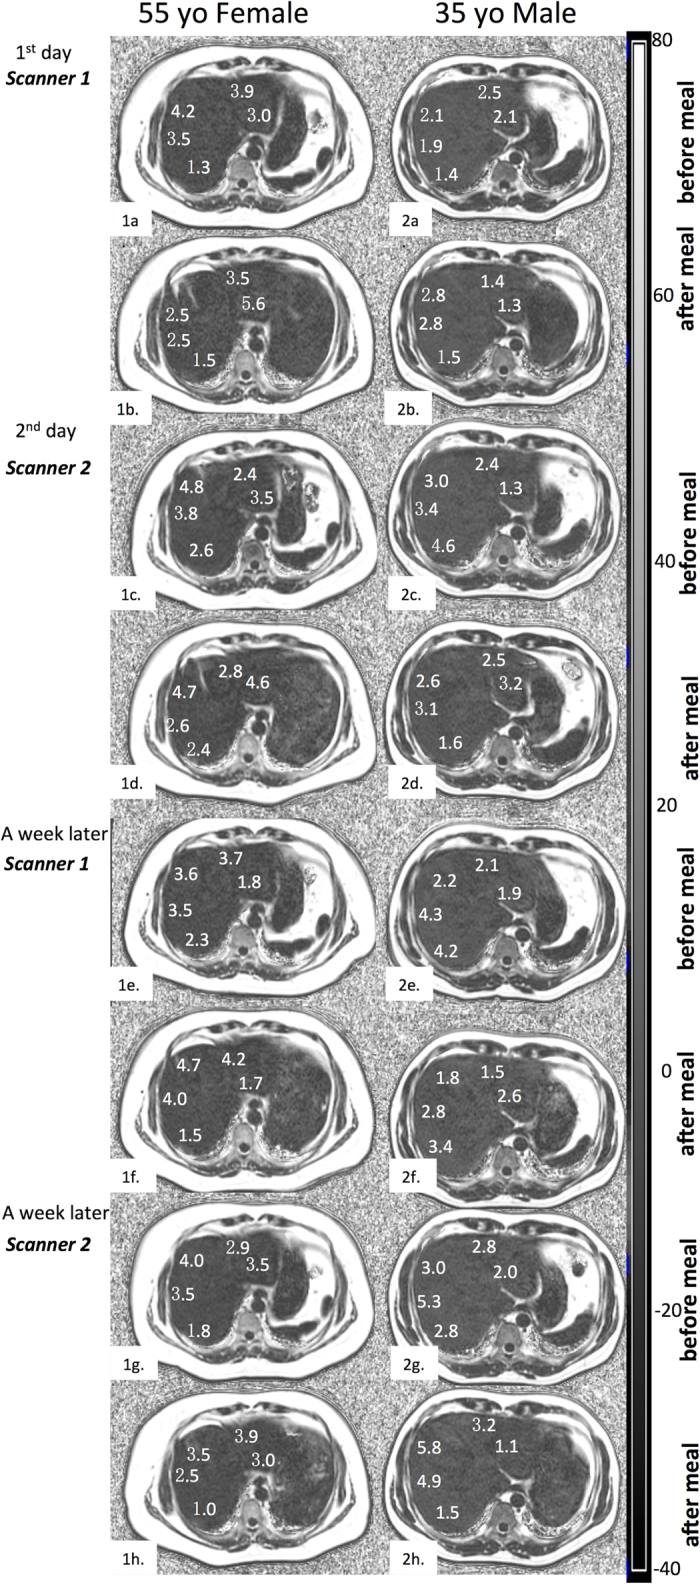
Representative transverse PDFF imaging (in units of %) obtained from two volunteers during eight tests on four respective days. PDFF maps were set with window level of 20 and window wide of 120. MRI-determined PDFF parametric maps of two subjects (55-year-old female, from 1a. to 1 h; 35-year-old male, from 2a to 2 h), demonstrating direct comparison of liver fat content for five ROIs placed on each liver during eight tests on four days. The close qualitative and quantitative agreements in MRI-determined PDFF on two 3.0-T scanners were recorded. The parametric maps were generated from source images by applying the pixel-by-pixel PDFF modelling algorithm of the commercial version. The parametric maps are displayed with a PDFF ranging from 0%–100%. Subcutaneous adipose tissue appeared to be white on the parametric maps because the fat fraction in the adipose tissue was approximately 100% PDFF.
